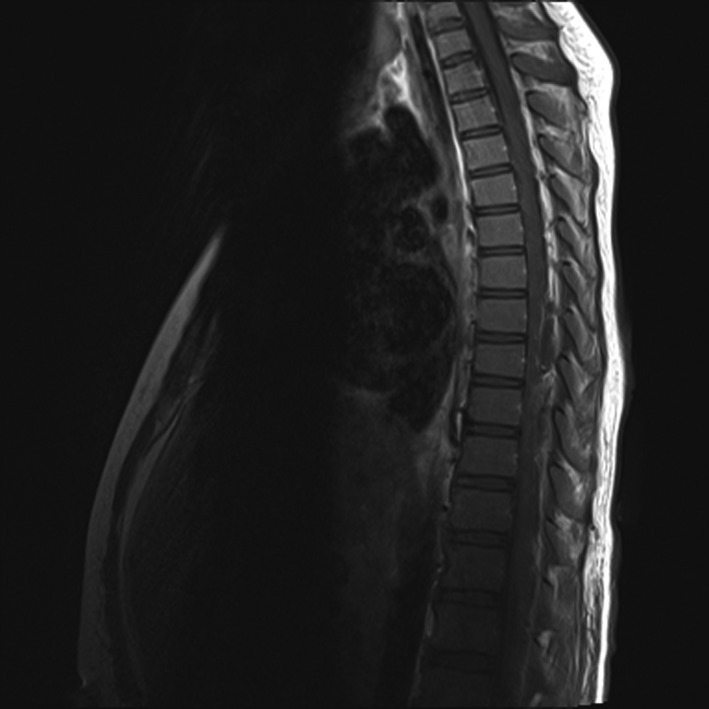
MRI T1 sagittal image is showing an iso‐intense lesion within the spinal canal spanning T7‐T10.
